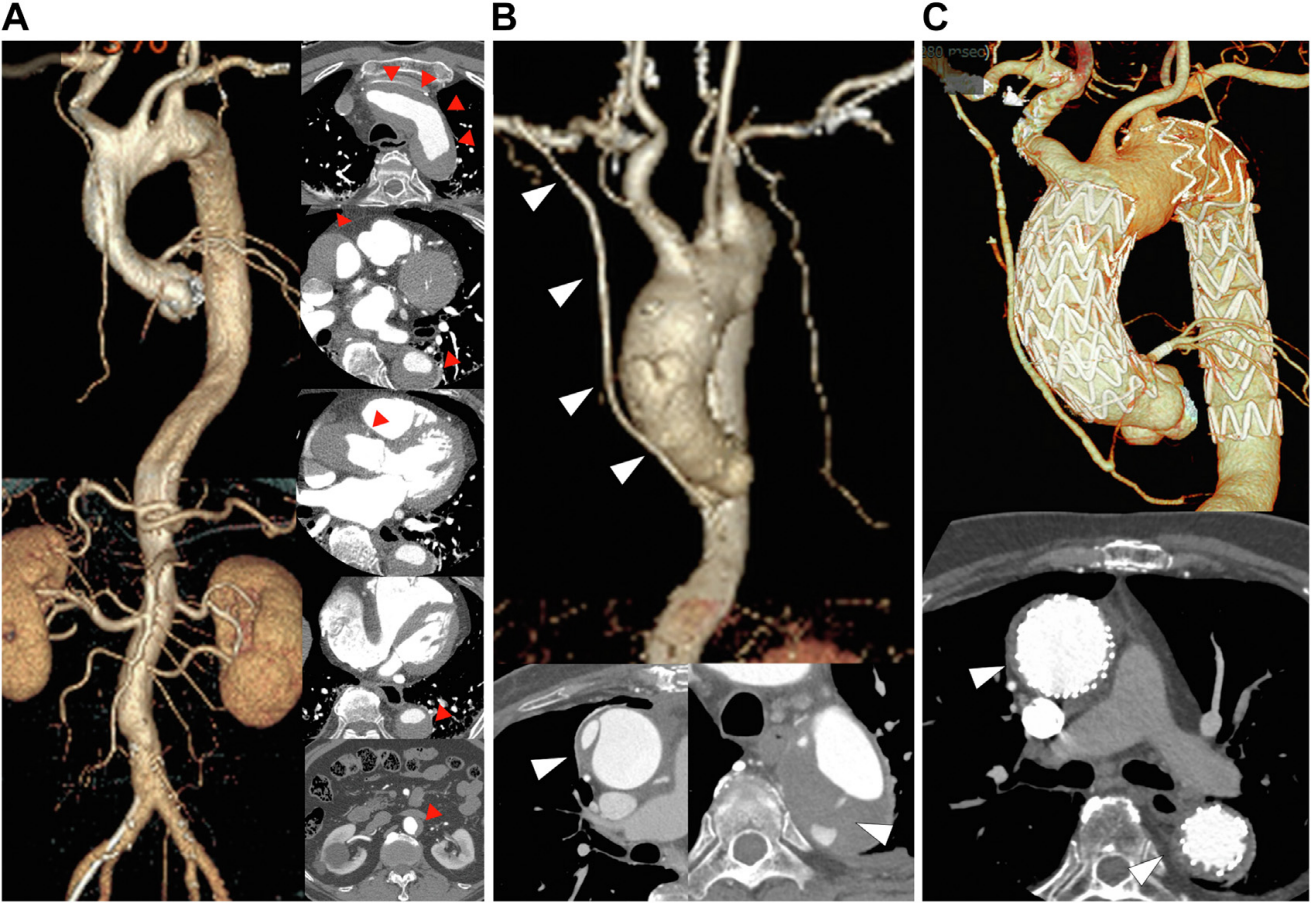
(A) Preoperative contrast-enhanced computed tomography (CT) scan (before coronary artery bypass grafting) showing false lumen thrombosis extending from the ascending aorta to the abdominal aorta, with right coronary artery occlusion (arrowheads). (B) Postoperative contrast-enhanced CT scan (2 months after coronary artery bypass grafting) showing patency of the saphenous vein graft, open false lumen of the ascending aorta, and thrombosed false lumen of the descending aorta. Tears in the ascending and descending aorta (arrowheads). (C) Postoperative contrast-enhanced CT scan (3 years after thoracic endovascular aortic repair) showing complete shrinkage of the false lumens in the ascending and descending aorta and enlargement of the true lumen (arrowheads).
